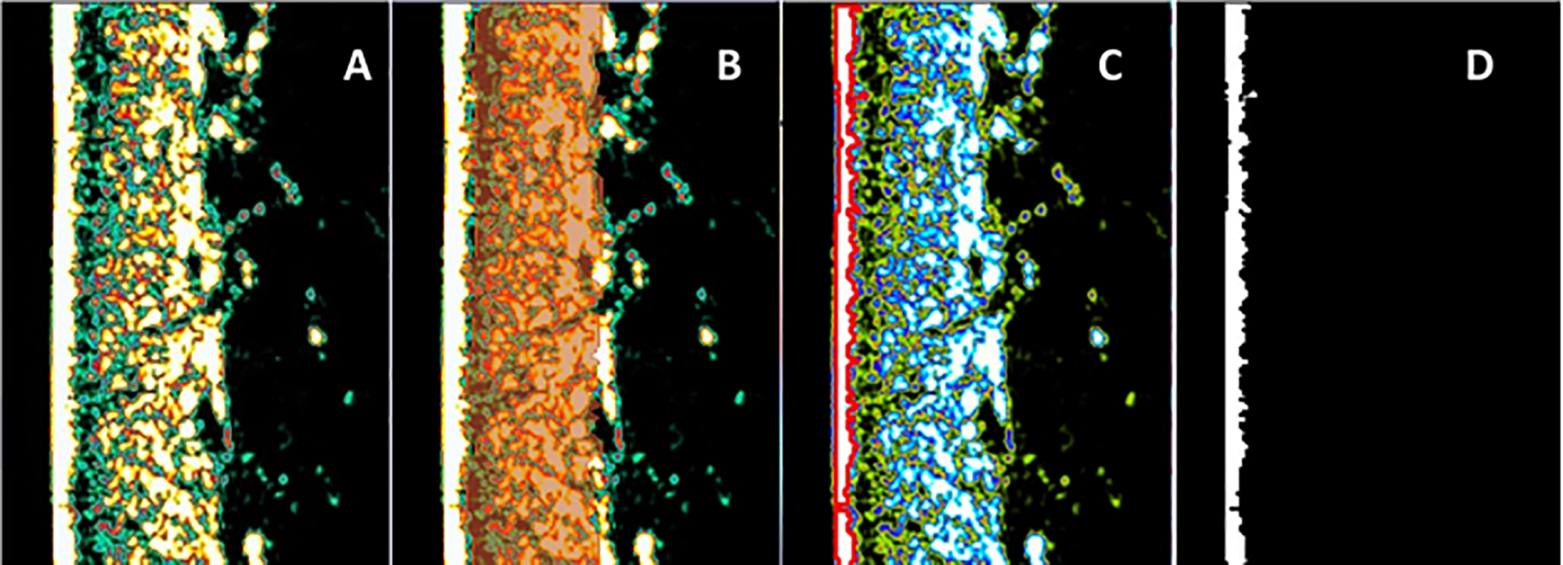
High-frequency ultrasonography image of the skin over the forearm with automated epidermal thickness estimation. (A) Skin ultrasonography image. (B) The red lines and the grid in between indicate the area over which dermal thickness is calculated using the DermaLab^®^ software. (C) The red color corresponds to the automated epidermal boundary detection by our dedicated software. (D) The white and black limits of the epidermis correspond to the automated mean thickness estimation by our dedicated software.
